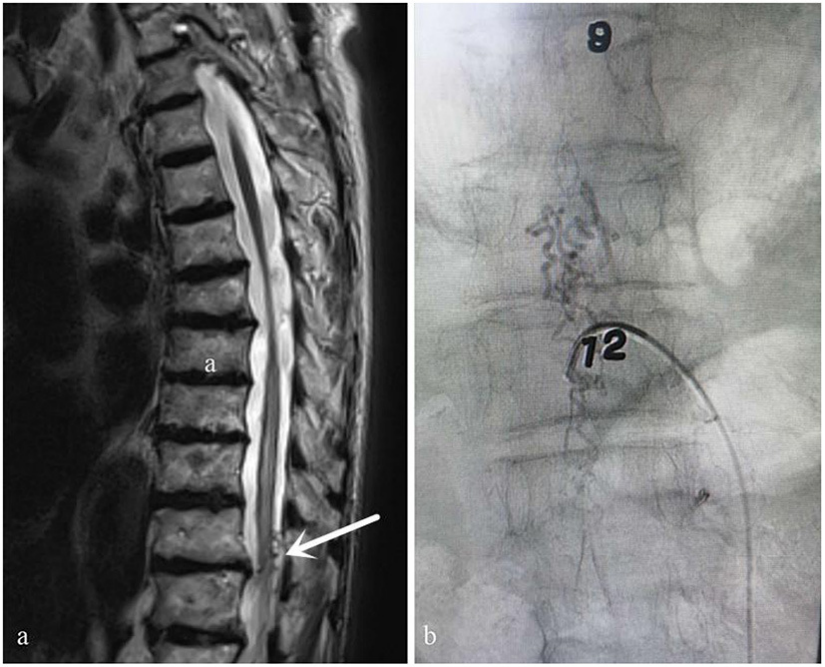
Case 2. A 73-year-old man who did not have typical signs of spinal cord edema and vessel flow voids on the cord on MRI (a) (white arrow: Atypical angiogram). This patient was misdiagnosed with thoracic spinal stenosis by an orthopedic surgeon. His symptoms were not completely consistent with the symptoms of thoracic spinal stenosis and worsened after he was treated for thoracic spinal stenosis. After MDT consultation, the diagnosis of spinal DAVF was confirmed *via* DSA performed by a neurosurgeon. The fistula was located at T9-10 (b).
